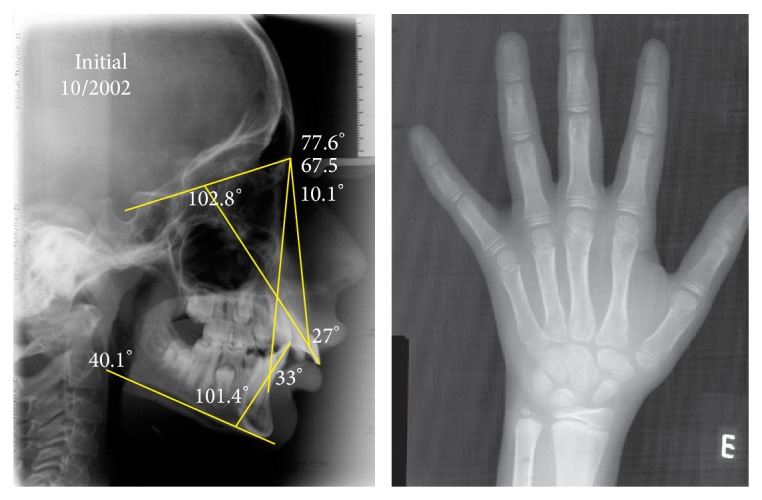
Pretreatment lateral cephalometric radiography; cephalogram; and hand-wrist radiography.
